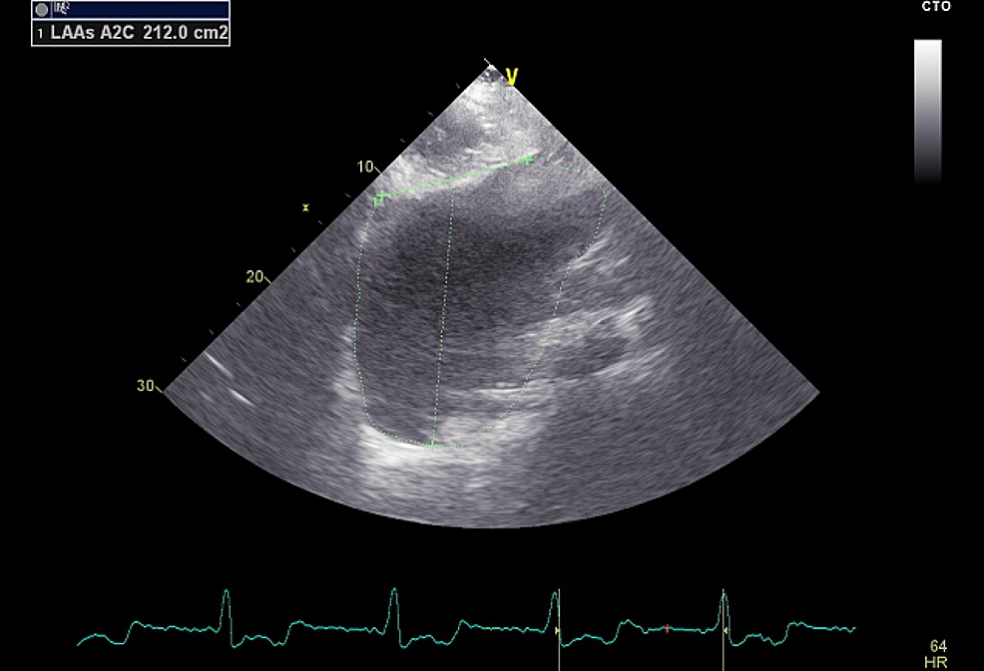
Echocardiography image of the left atrium (LA volume)
